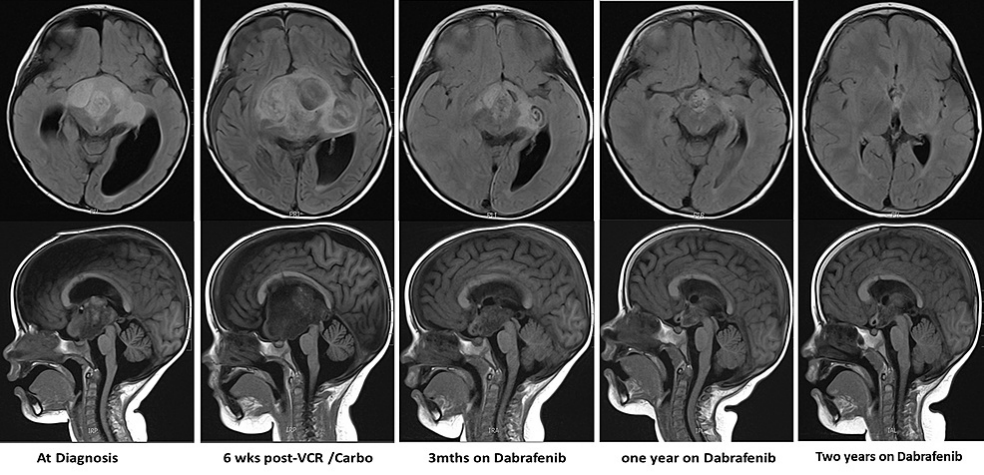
Longitudinal neuro-surveillance with MRI of our patient. Longitudinal surveillance neuroimaging showing T-1 FLAIR MRI images (upper panel: axial; lower panel: sagittal) at various stages of management of the patient. VCR, vincristine; Carbo, carboplatin; FLAIR, fluid-attenuated inversion recovery
